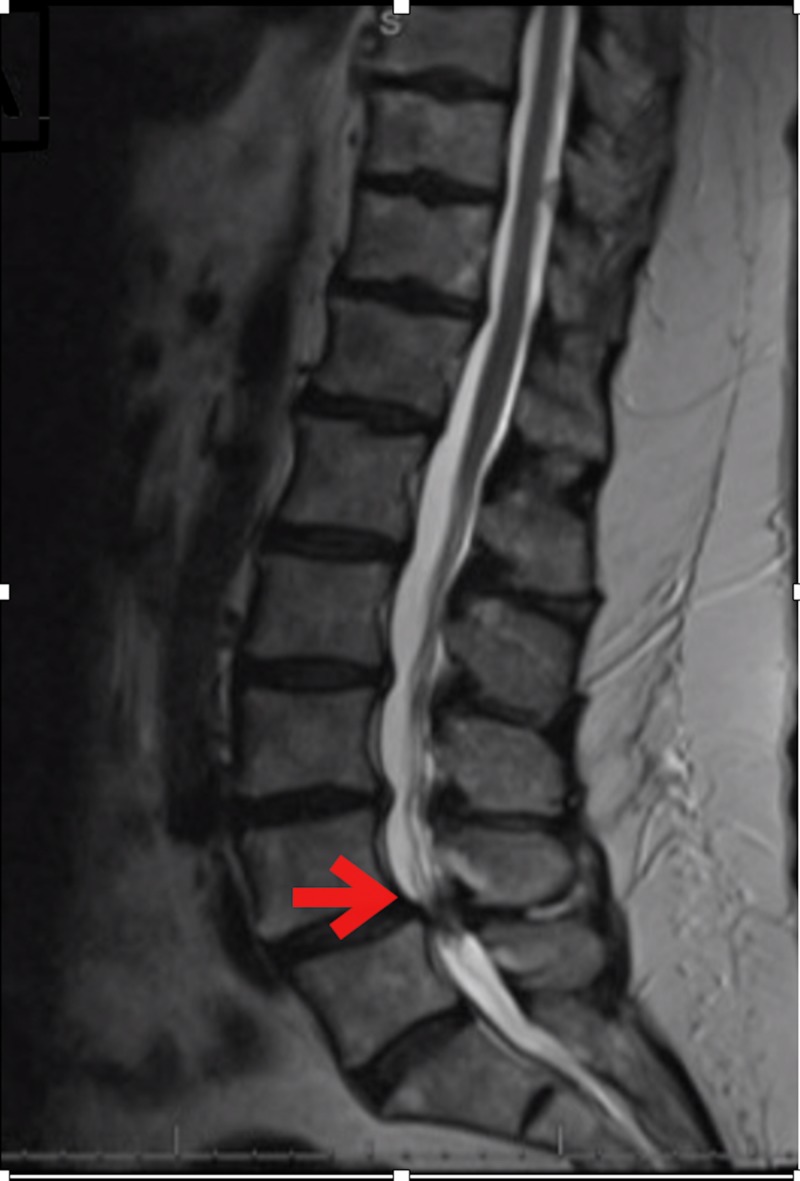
Pre-operative sagittal T2-weighted MRI demonstrating grade 1 spondylolisthesis at L4/5 with severe canal stenosis.
